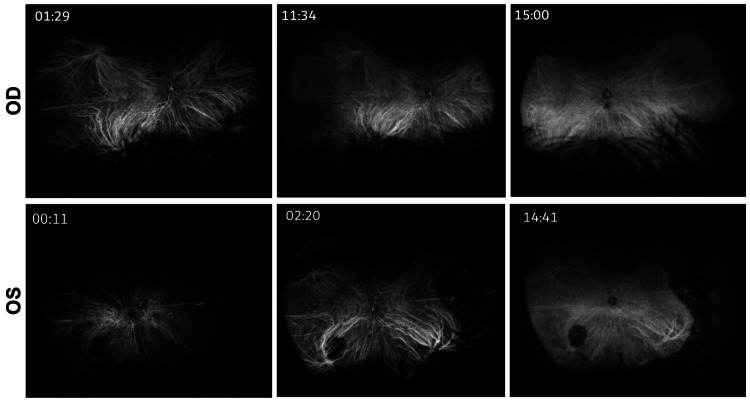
Serial indocyanine green angiography findings at follow-up (2024 visit) Indocyanine green angiography obtained in the early, mid, and late phases. Top row (OD): no major abnormality. Bottom row (OS): multiple hypocyanescent choroidal lesions/spots corresponding to the affected areas, with persistent abnormalities across sequential phases. These findings provided additional evidence of choroidal involvement and complemented the fundus, optical coherence tomography, fluorescein angiography, and ultrasonographic findings. OD, oculus dexter (right eye); OS, oculus sinister (left eye)
